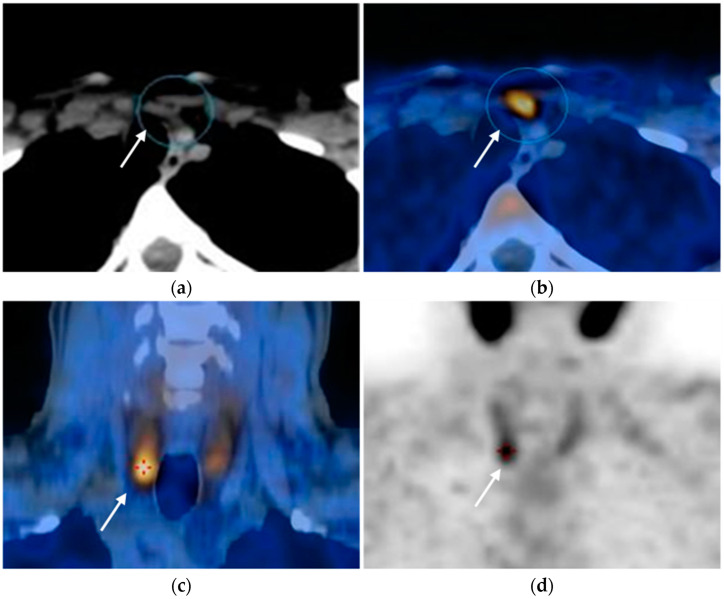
Parathyroid adenomas. 18F-fluorocholine PET/CT (a,b) and parathyroid scintigraphy (c,d) of two different patients show radiotracer uptake within parathyroid glands (arrows).
